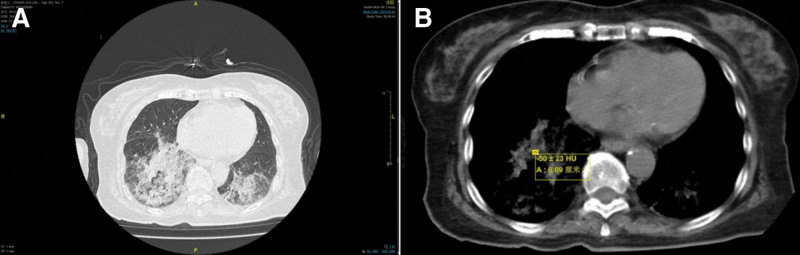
Chest computed tomography shows bilateral pneumonia lesions, manifested as ground glass opacities in the middle lobe of the right lung and lower lobes of both lungs, consolidation shadows, and thickening of interlobular septa (crazy paving) (May 04, 2024).
